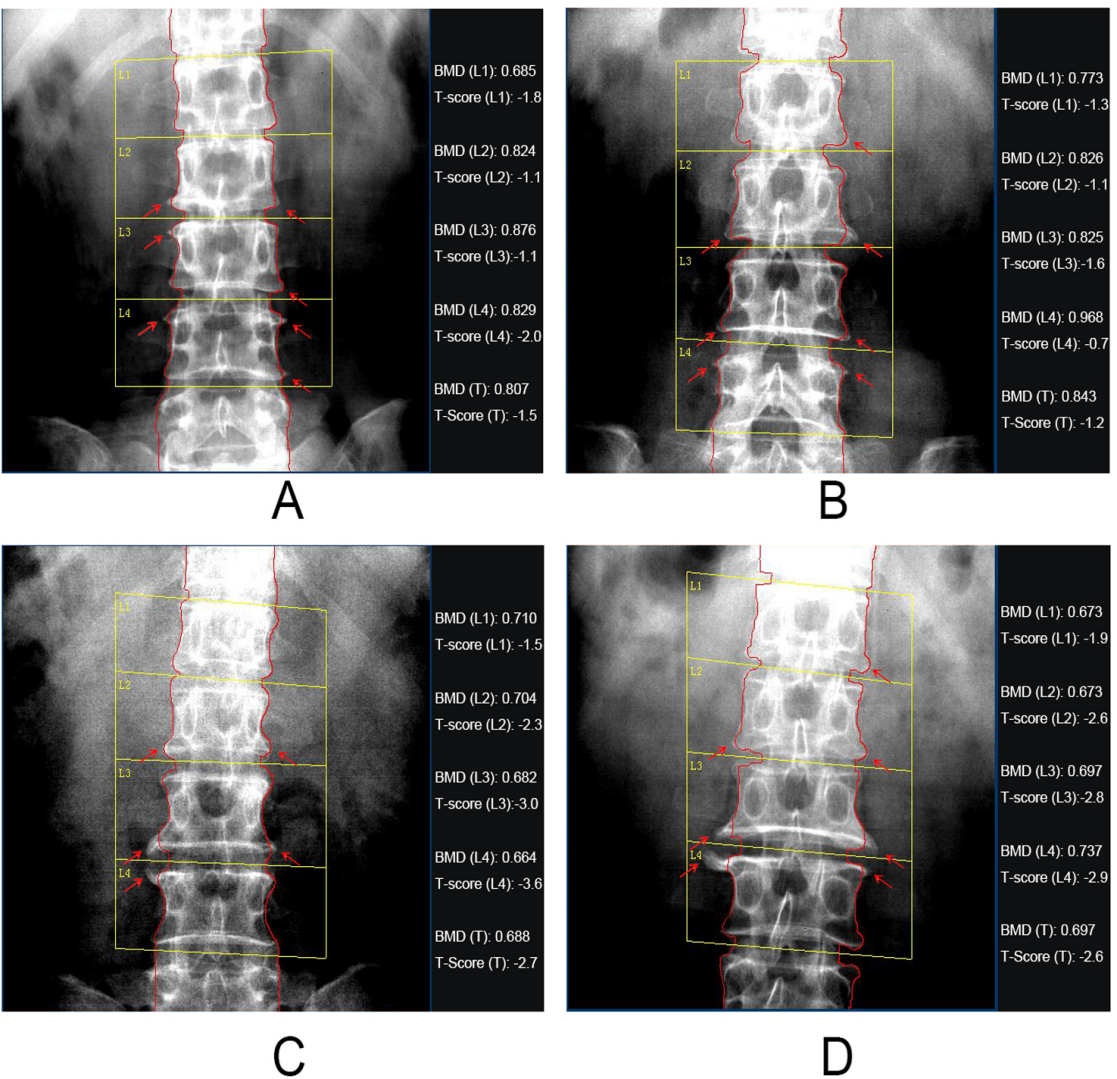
Participants’ X-ray films of lumbar vertebrae. 4 participants were selected. Two participants, A and B were patients with BMD decrease. The other two participants, C and D, were patients with osteoporosis. X-ray films were shot by LEXXOS dual-energy X-ray absorptiometry. The status of BMD in the lumbar vertebrae of participants, including the respective and total (T) actual measured value and corresponding T value (T—score) of BMD in their lumbar L1-L4, was marked on the right side of each X-ray film. The red contour lines around vertebral body indicate the outer contour of vertebral body when measuring BMD, and the red arrows indicate the status of bone spur formed by the participants’ hyperostosis.
